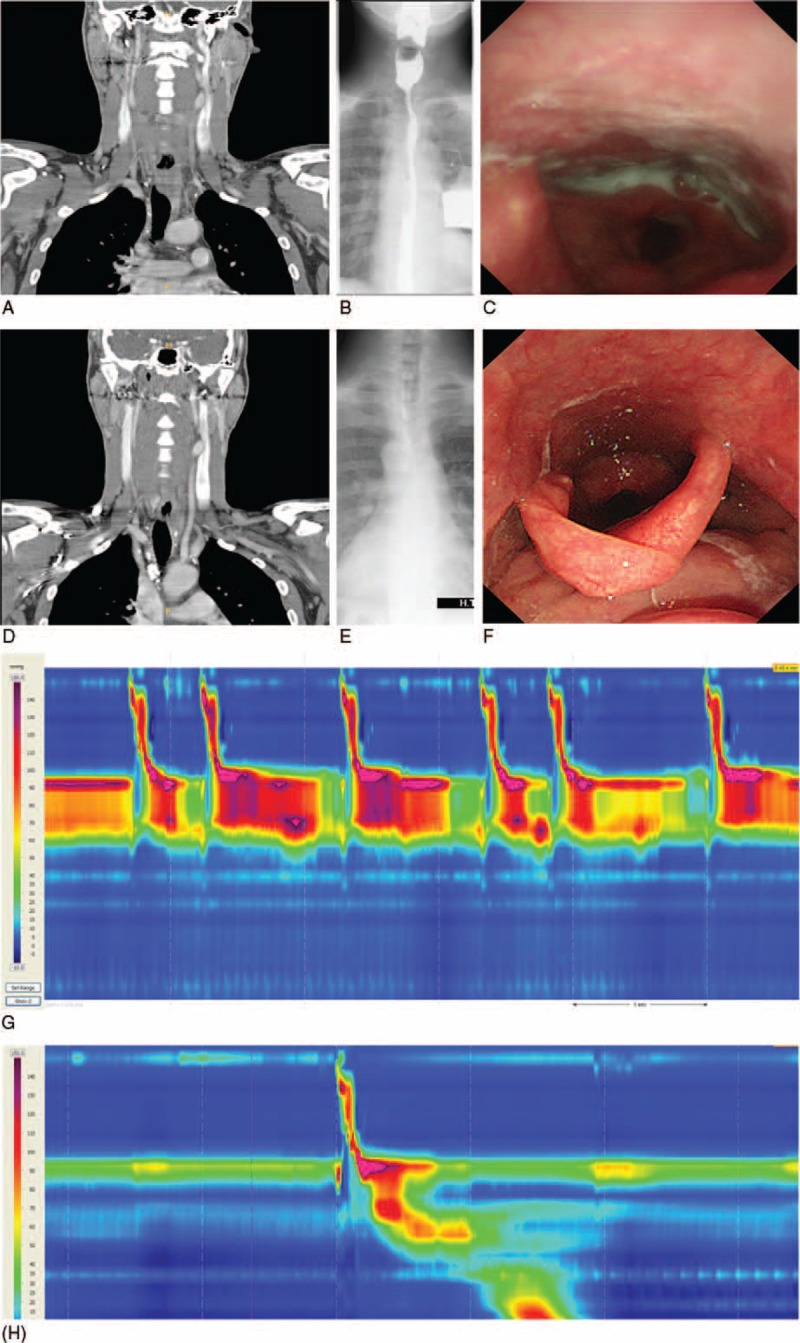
Neck computed tomography, gastrografin esophagography, flexible endoscopic evaluation of swallowing, and high-resolution manometry (A–C, G) before and (D–F, H) after treatment with prednisolone. (A) An approximately 3.9-cm mass in the esophagus causing mild indentation and shifting of the trachea. (B) Dilated pharyngeal cavity with a large amount of residue at both pyriform sinuses. (C) Severe amount of residue at both pyriform recesses. (D) Decreased size of ill-defined mass surrounding lower cervical esophagus and improved indentation of abutting trachea. (E) Near resolution of upper esophageal stricture. (F) No residue at either of the pyriform recesses or the valleculae. (G) Repetitive swallowing and hypercontraction just below the upper esophageal sphincter 11 months after treatment. (H) Complete disappearance of the hypercontraction just above the upper esophageal sphincter and appearance of normal upper esophageal contraction.
